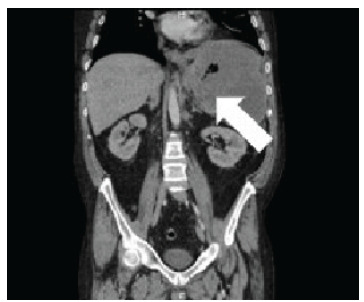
Computed tomography abdomen and pelvis coronal image showing the presence of gas (arrow) within the spleen.
